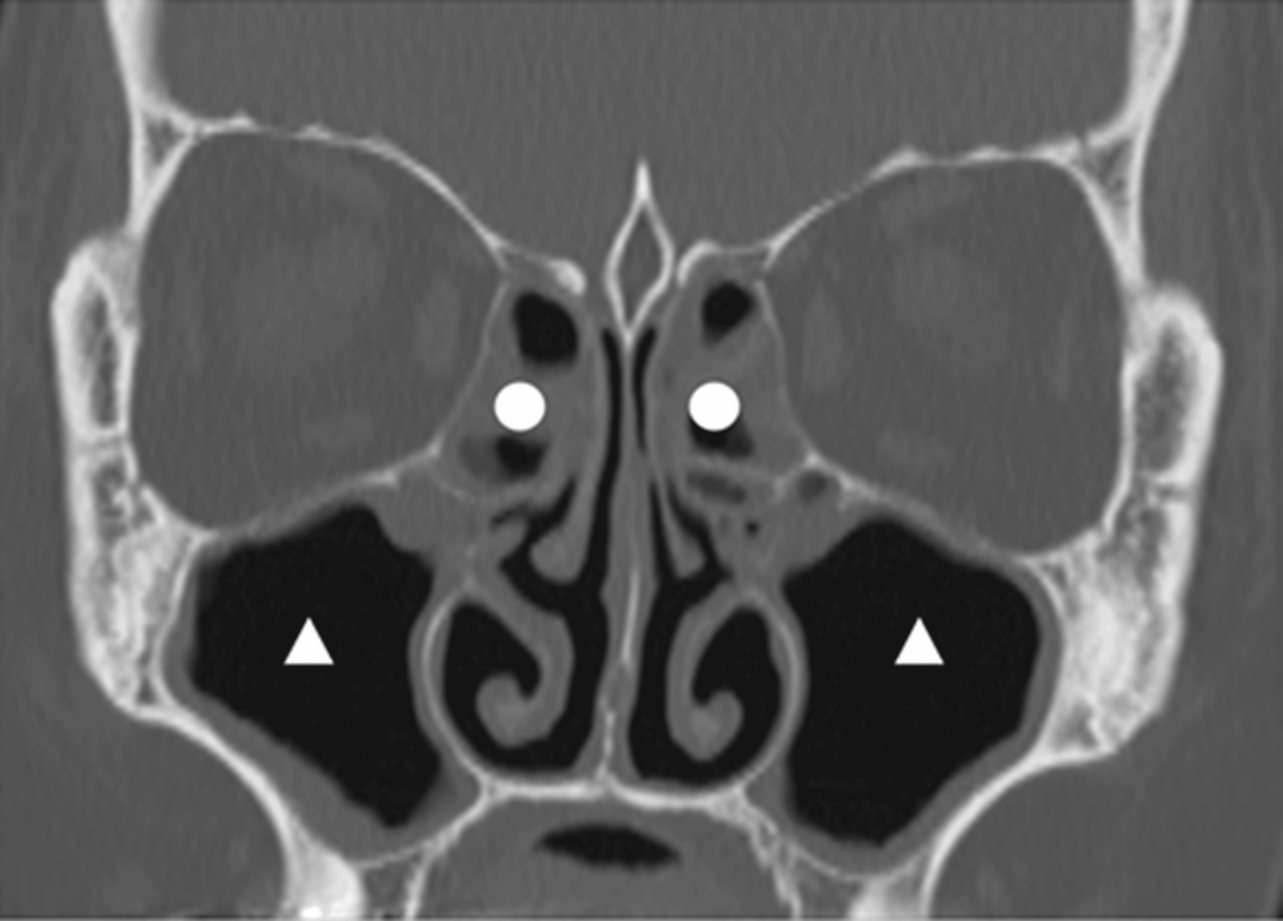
Sinus CT (coronal section) Opacities were more prominent in the ethmoid sinuses (〇) than in the maxillary sinuses (△).
